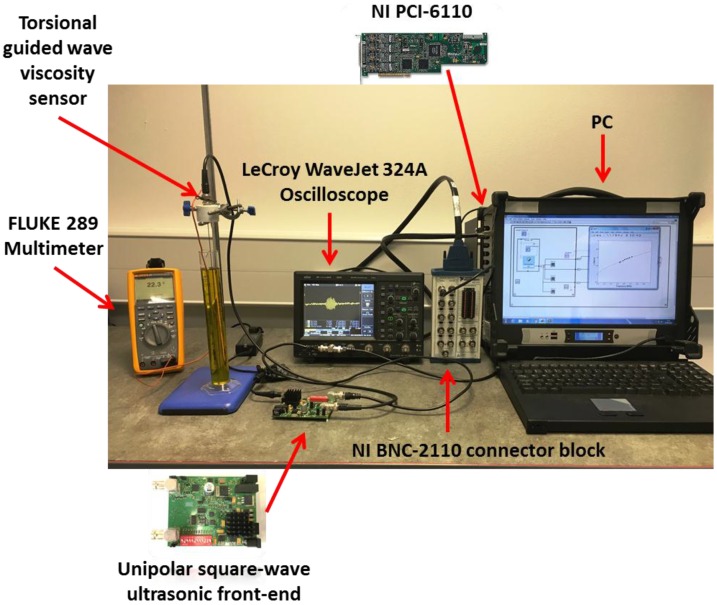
Unipolar ultrasonic square wave front-end experimental setup for torsional guided wave viscosity sensor.
